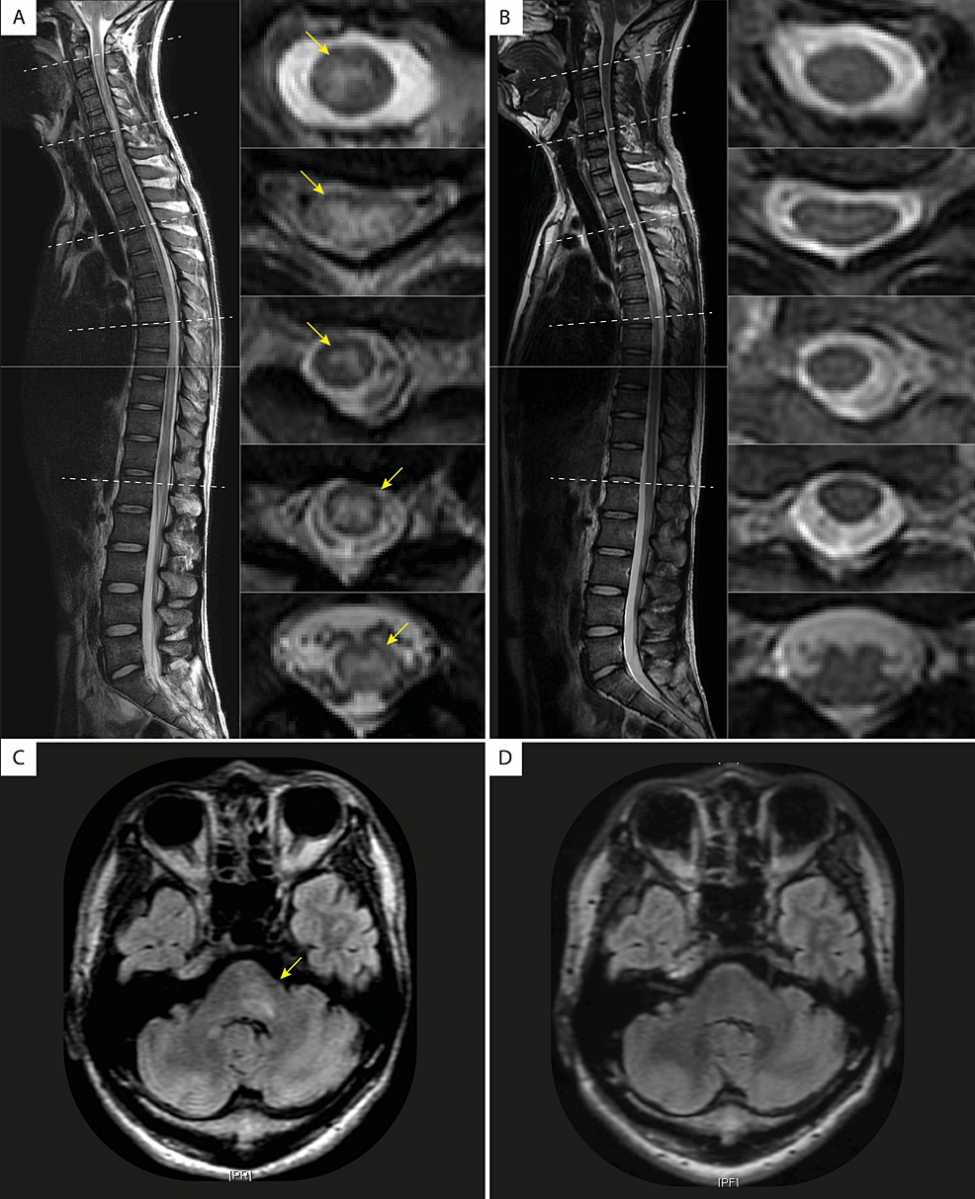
Radiological findings of the patient before and after treatment. A) Saggital and axial views of T2-weighted MRI of the spinal cord before treatment showed a high-intensity lesion ranging from C2 to the conus. B) Saggital and axial views of T2-weighted MRI 28 days after admission showed complete resolution of high-intensity lesions. C) Fluid attenuated inversion recovery (FLAIR) sequence of the axial view of the brain MRI before treatment showed a high-intensity lesion in the left side of the pons (arrowhead). D) The left pontine lesion was not evident in the FLAIR sequence of the axial view of the brain MRI after treatment.
